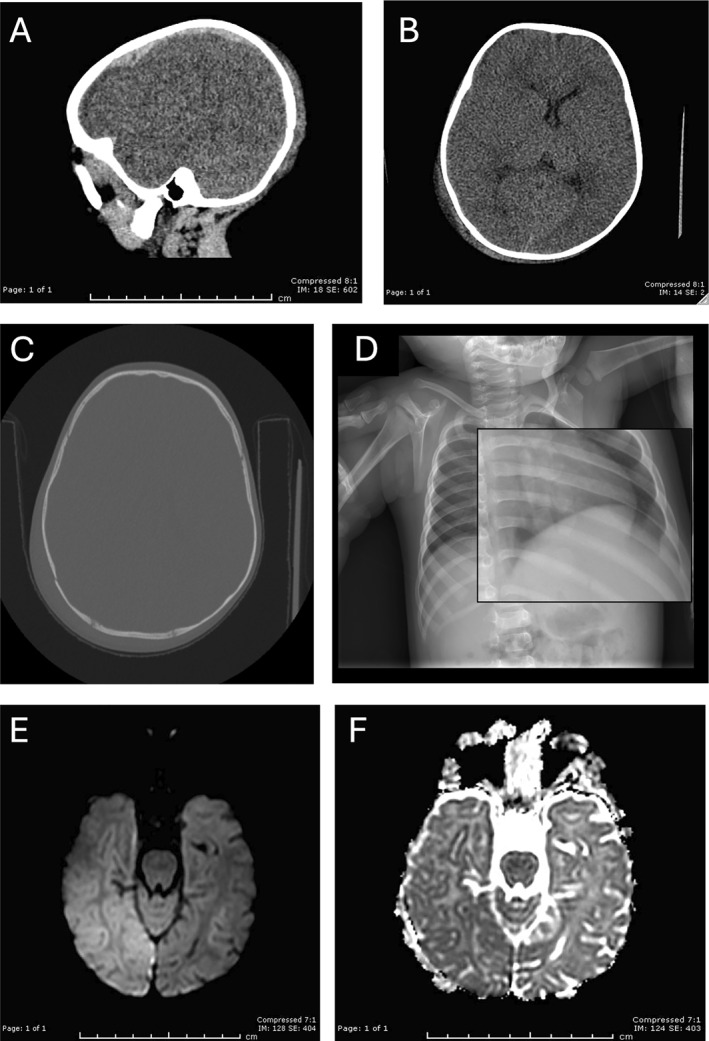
An 8‐month‐old male with a reported unwitnessed fall and bilateral retinal hemorrhages who presented before the pandemic. (A–C) CT axial images demonstrate an acute subdural hematoma with extension along the falx, left to right midline shift, and left‐sided cerebral edema in association with a calvarial fracture involving the right parietal bone with overlying scalp hematoma, (D) the skeletal survey demonstrates periosteal reaction along the left seventh rib posteriorly consistent with healing fracture, and (E and F) MRI diffusion‐weighted images demonstrate infarction of the right cerebral hemisphere.
